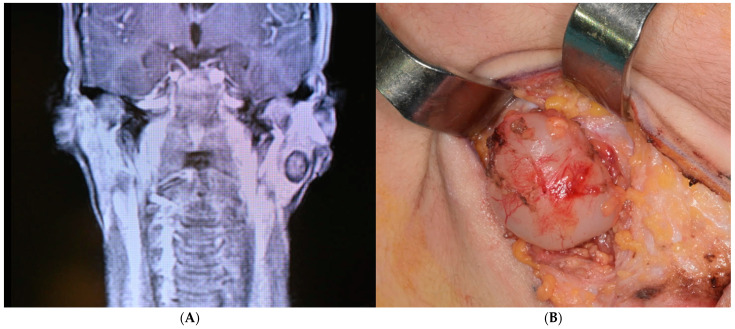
(A): MRI image of the tumor. (B): appearance of the lesion at surgery.
